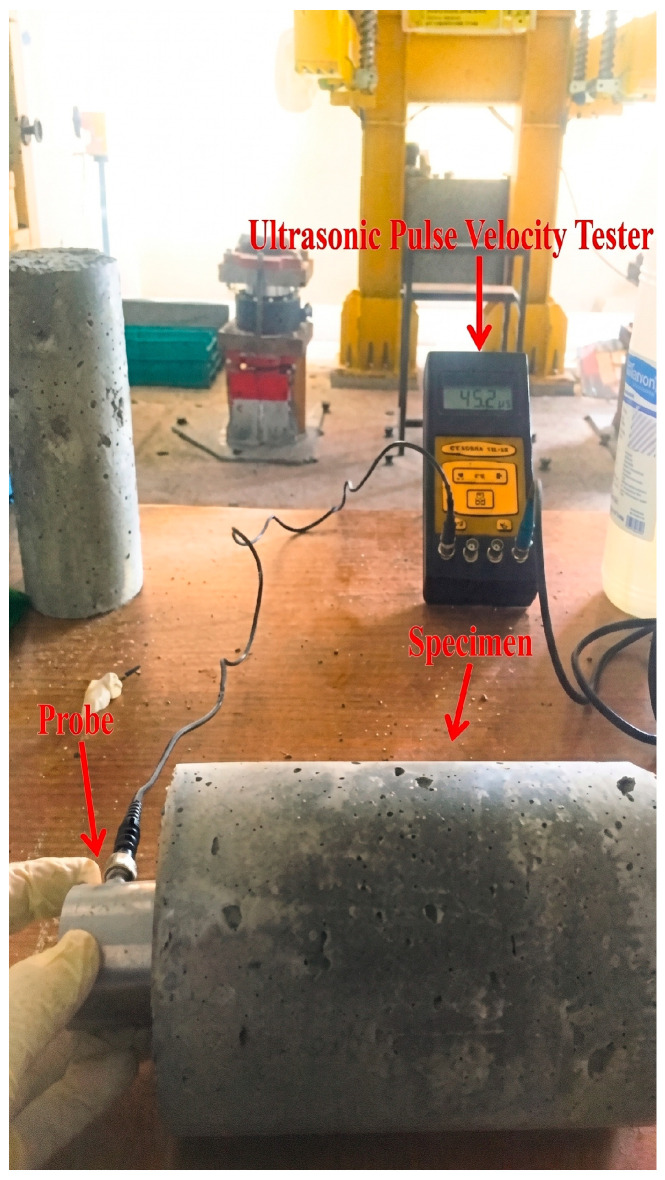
Ultrasonic pulse velocity.
